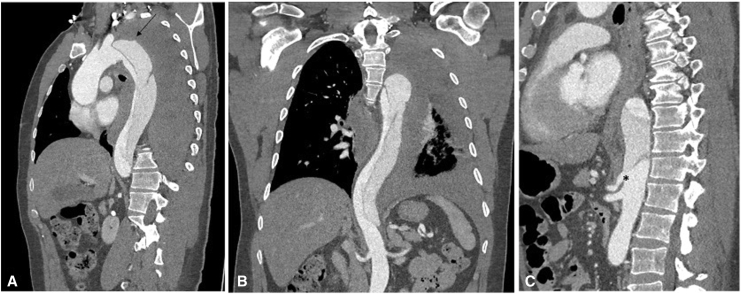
CT aortography in complex type B aortic dissection. Oblique sagittal CT reformat (A) shows complex dissection flap distal to the origin of the left subclavian artery, with the smaller true lumen pushed to the anteromedial aspect of the arch of aorta and descending thoracic aorta by the larger false lumen, which shows faint contrast opacification. Note the left hemothorax (attenuation 45 HU) and the irregular contour of the false lumen superiorly (*black arrow*), suggesting ruptured dissection. Coronal oblique (B) and sagittal oblique (C) CT reformats show the distal extent of the dissection, reaching just above the celiac axis origin (*asterisk*). *CT*, Coronary angiography.
